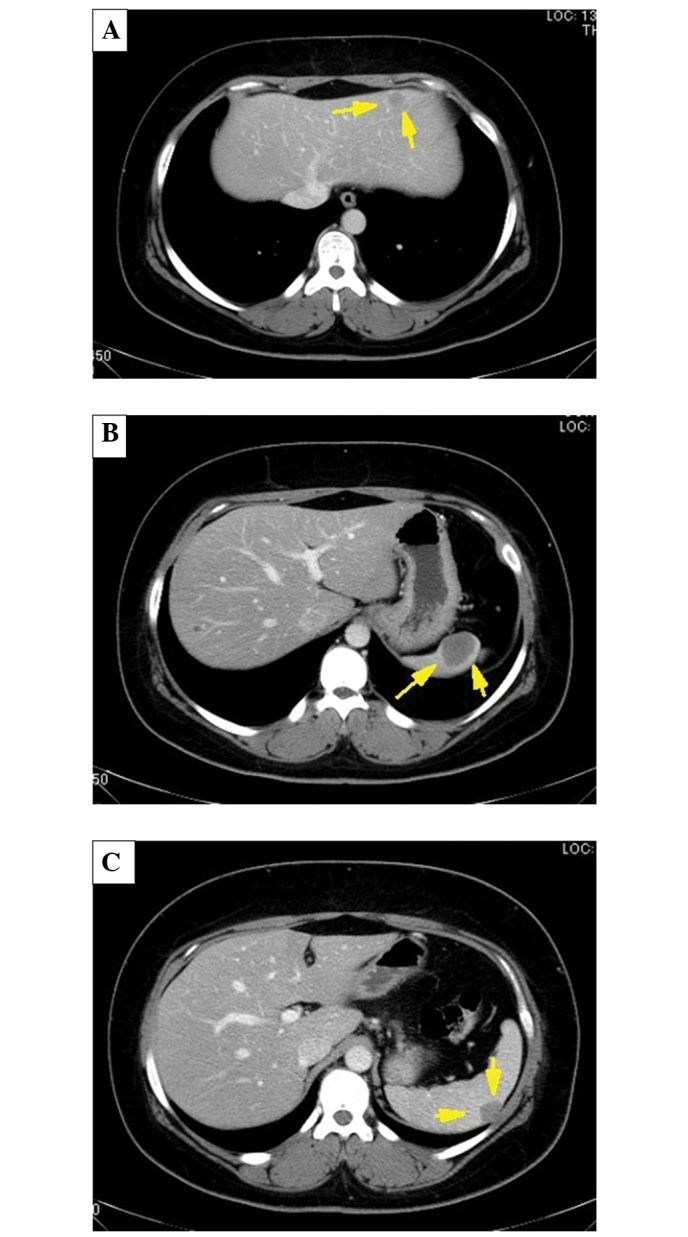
Contrast-enhanced computed tomography, which was performed again prior to surgery, revealing (A) a low-attenuation nodule (arrows) in the lateral segment of the liver and (B and C) two low-attenuation nodules (arrows) in the spleen.
